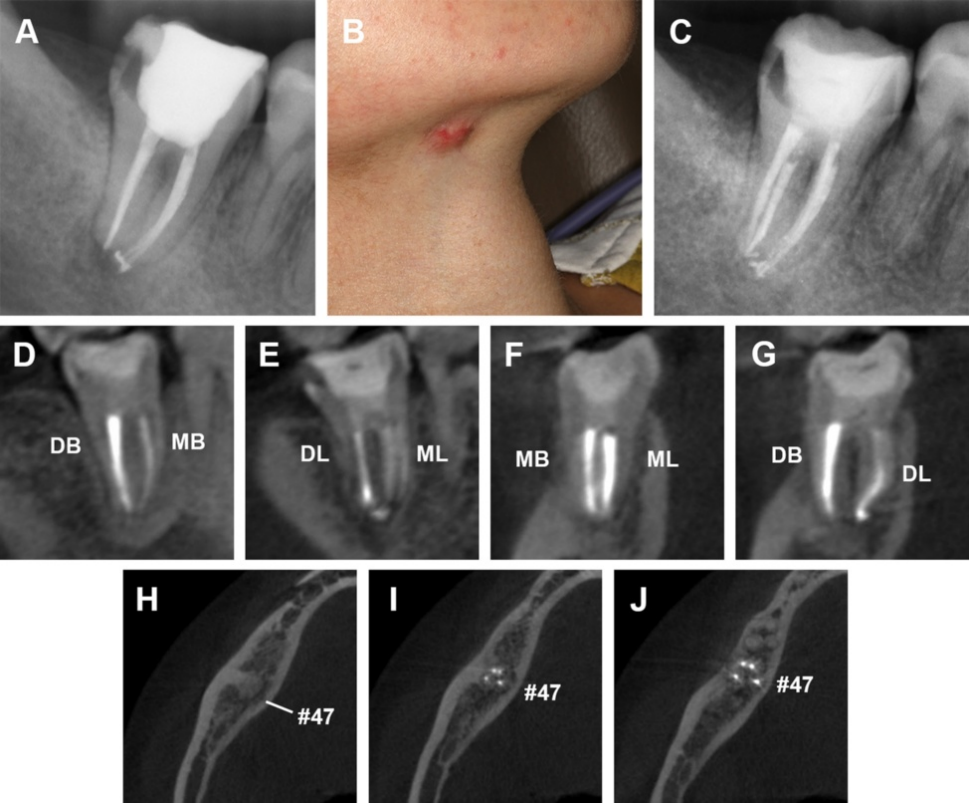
Postoperative radiographs of endodontic treatment and follow-up after five and nine months. (A) Post treatment periapical radiograph revealing that the obturation is of good quality. At the five-month follow-up, the extra-oral view (B) shows that the sinus tract had disappeared but left a scar in the right mandibular region. The periapical radiograph (C) shows the healing of periapical tissues. After nine months, postoperative CBCT images of the sagittal (D and E), coronal (F and G) and axial view (H-J)view confirmed the apical healing and adequate obturation. DB, distobuccal; MB, meisobuccal; DL, distolingual; ML, meisolingual.
